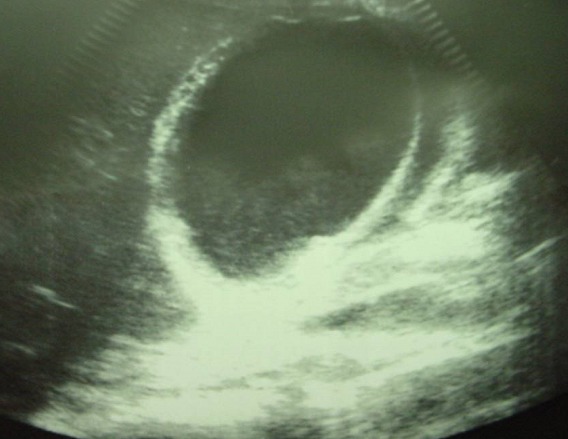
Ultrasonographic image of the gallbladder. Note the distension of the gallbladder, thickening of the wall, pericholecystic fluid collection, and sludge
